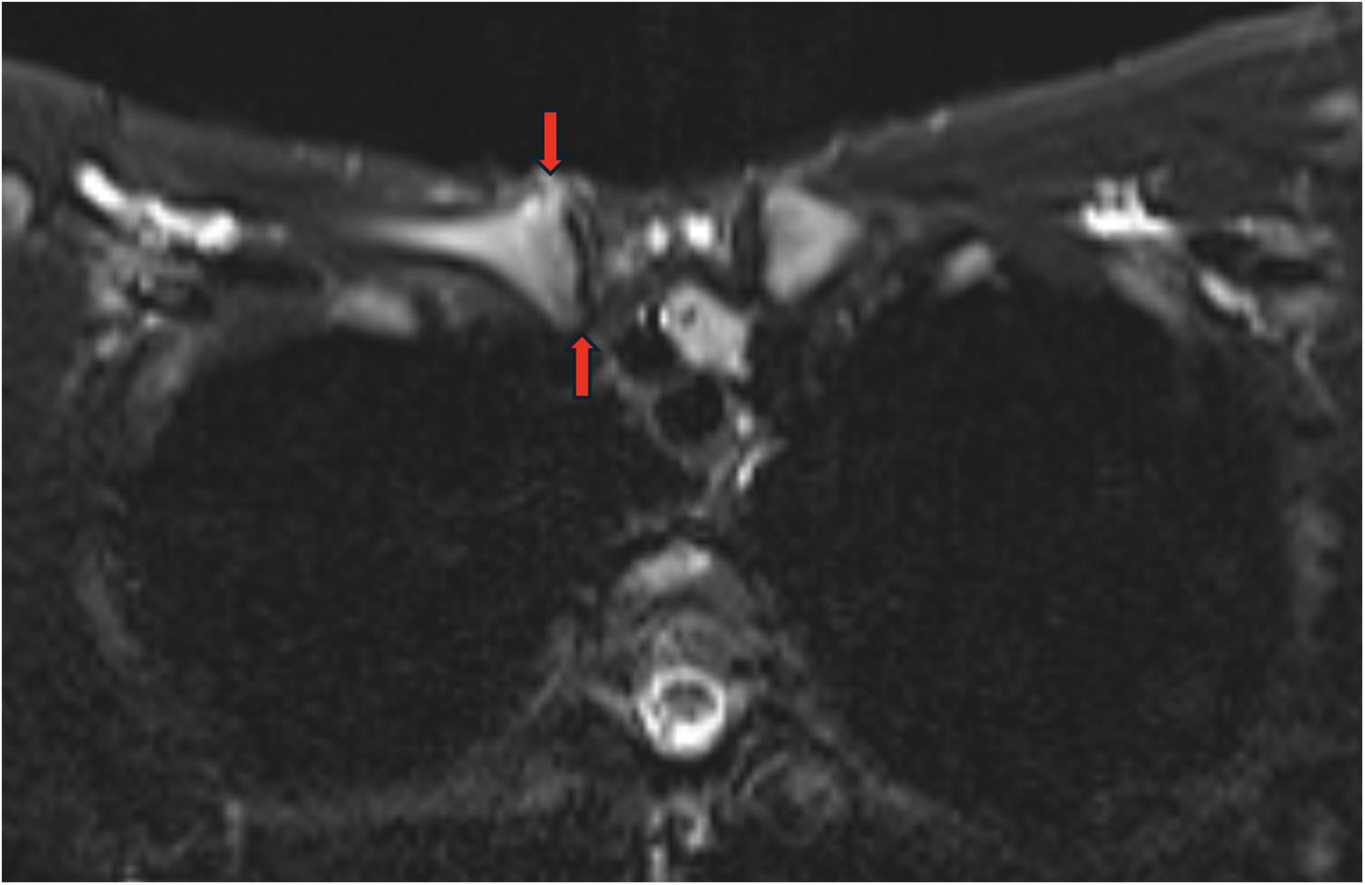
MRI showing a widening of the articular facet without erosion or signs of bone oedema (red arrows).
